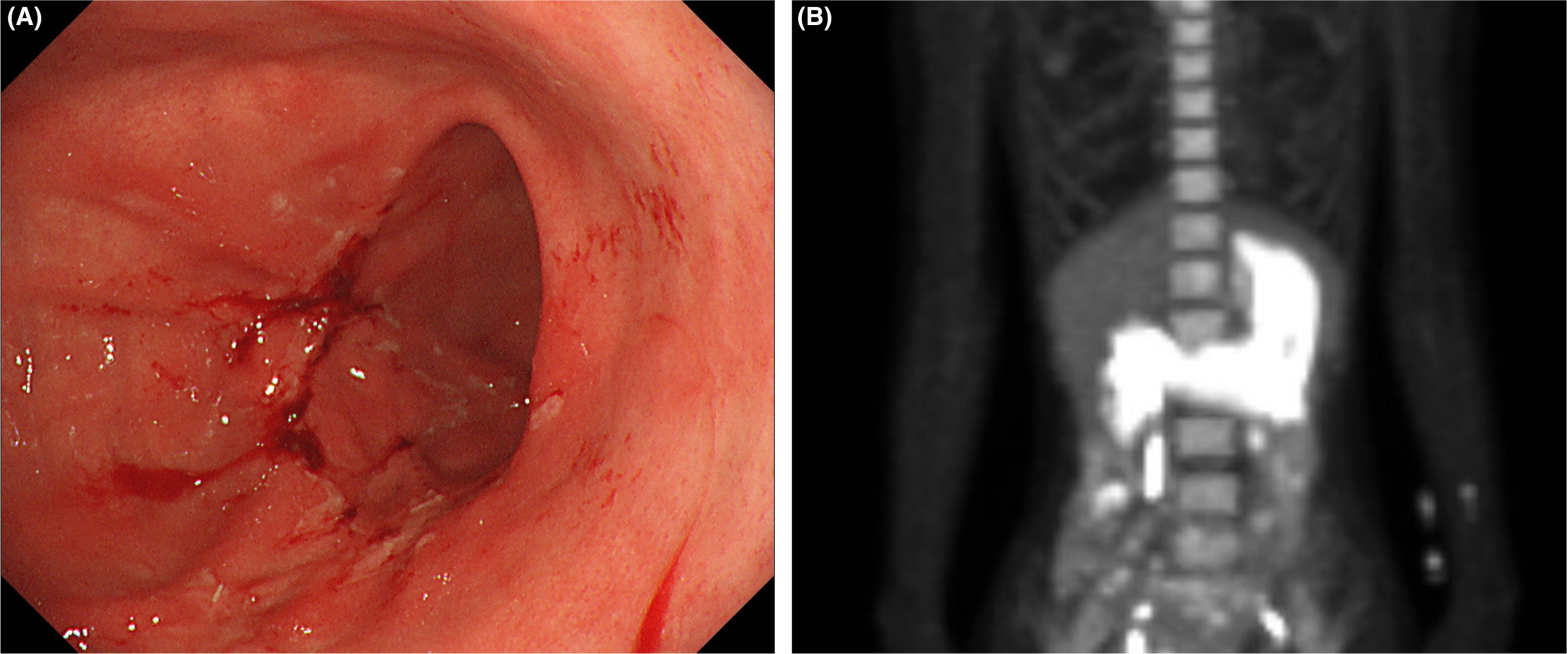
(A) Upper gastrointestinal endoscopic image. Gastric ulcers are seen on the anterior and posterior walls of the lower part of the gastric body. (B) FDG‐PET image reveals intense uptake by the entire gastroduodenal wall. Abbreviations: FDG‐PET, ^18^F‐fluorodeoxyglucose positron emission tomography
